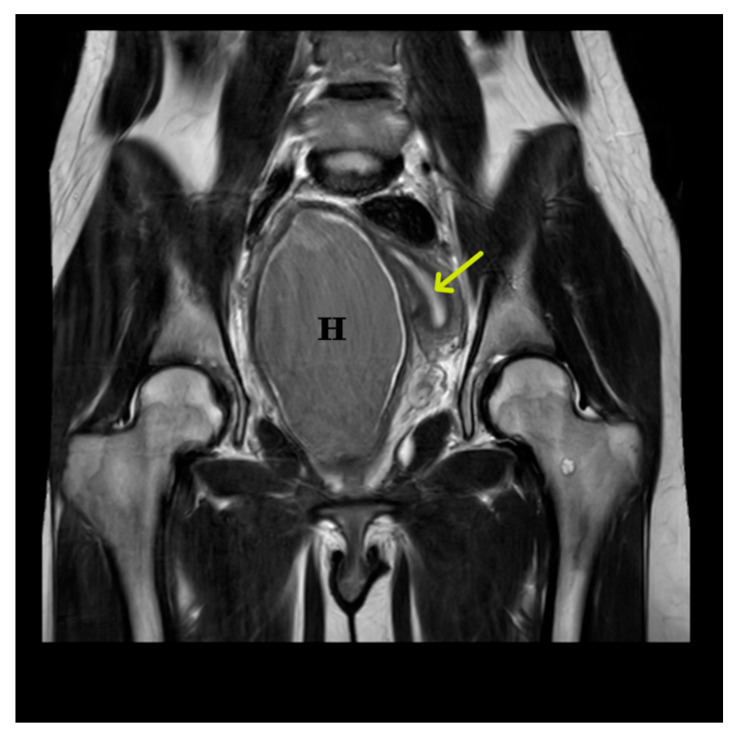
Preoperative coronal T2-weighted MRI of the pelvis, acquired prior to surgical incision of the hematocolpos. The examination was performed using a standard pelvic MRI protocol. The image demonstrated a markedly dilated right hemivagina containing material with intermediate-to-low T2 signal, consistent with hematocolpos (H), measuring approximately 68 × 77 × 120 mm (estimated volume ~330 mL). Both vaginal canals converged at the level of the vestibule. The vaginal septum was presumed to be longitudinal; however, its evaluation was limited by the presence of hematocolpos (H). Uterus didelphys was present, with two distinct uterine bodies: the right uterine body was superficially located in the right pelvic fossa, whereas the left uterine body was situated deeper and more posteriorly within the pelvis (yellow arrow). The urinary bladder and the visualized portion of the colon showed no apparent pathological changes.
